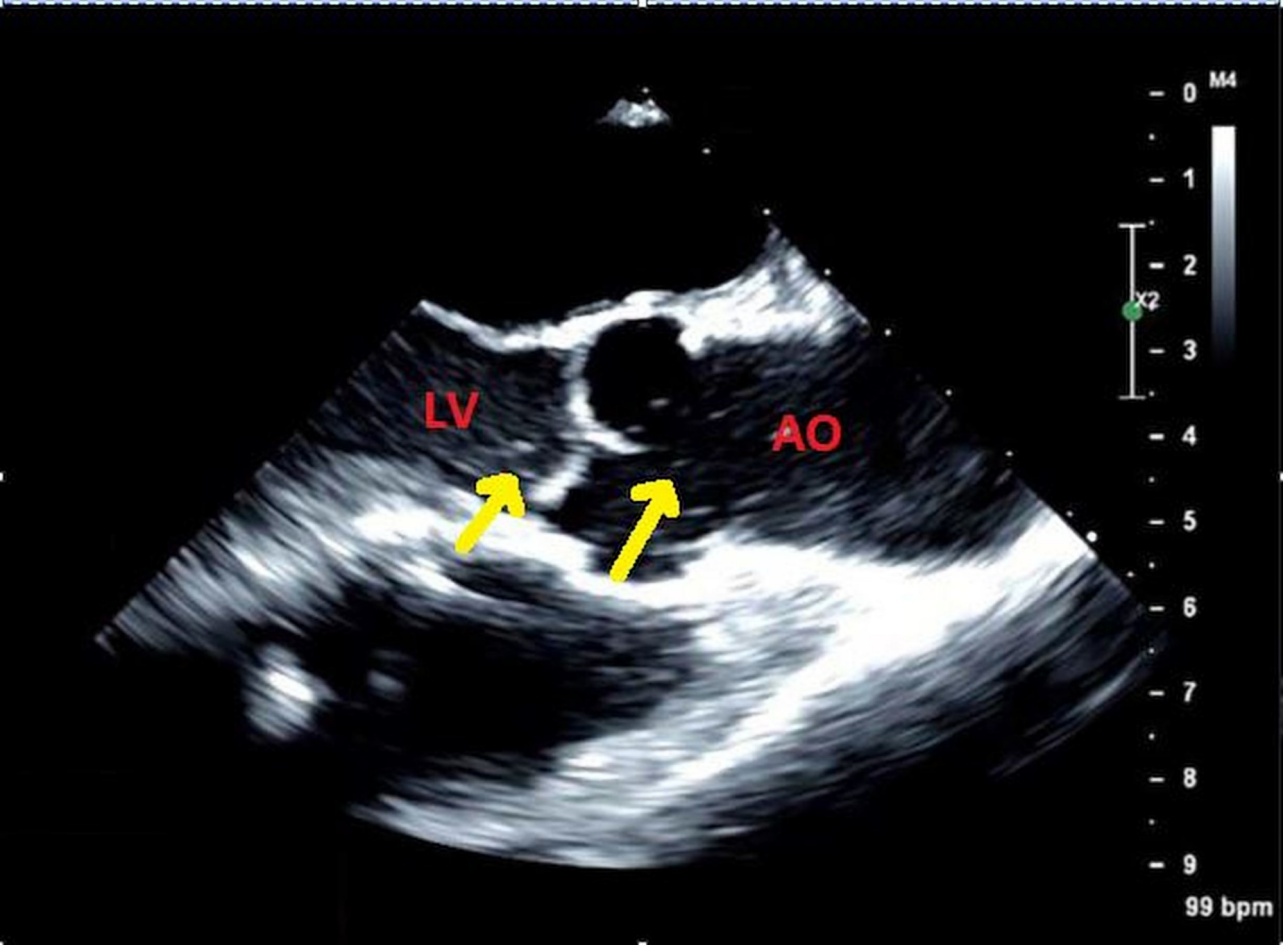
Intraoperative transesophageal echocardiogram before pump exchange showed microbubbles (arrows) in the aorta and left ventricle LV: left ventricle; AO: aorta
